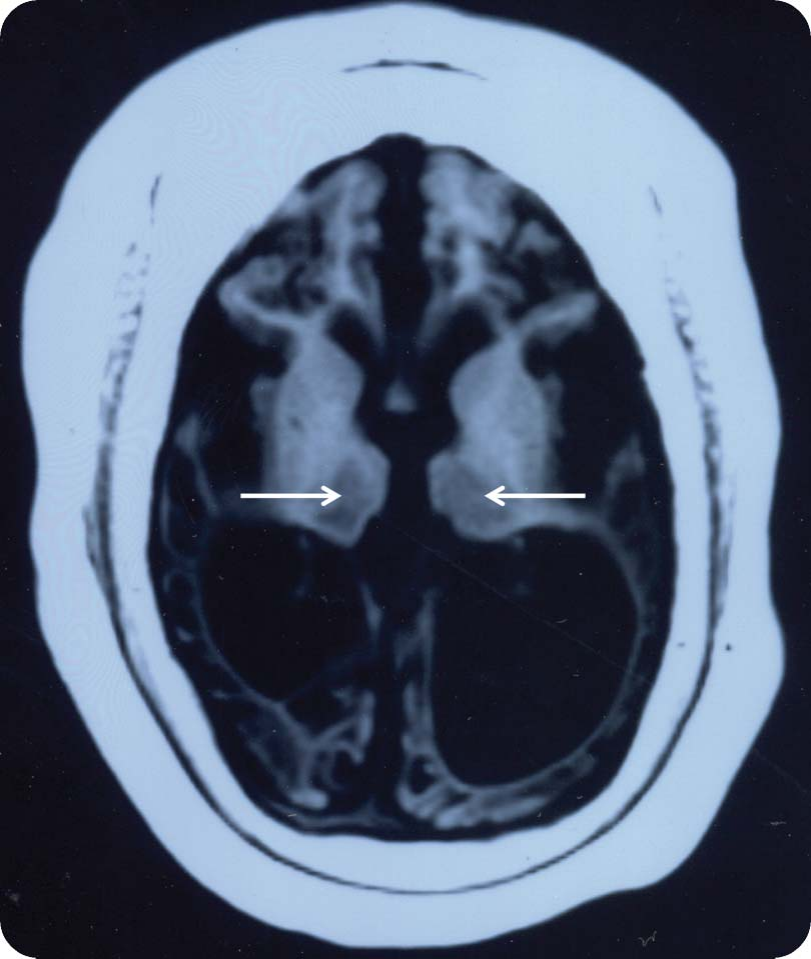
Brain imaging findings in the patient An axial T1-weighted MRI image of the patient at the age of 11 years. There is extensive loss of both gray and white matter with corresponding widening of the CSF spaces and thickening of the skull. Both thalami (arrows) show shrinking and signal change.
